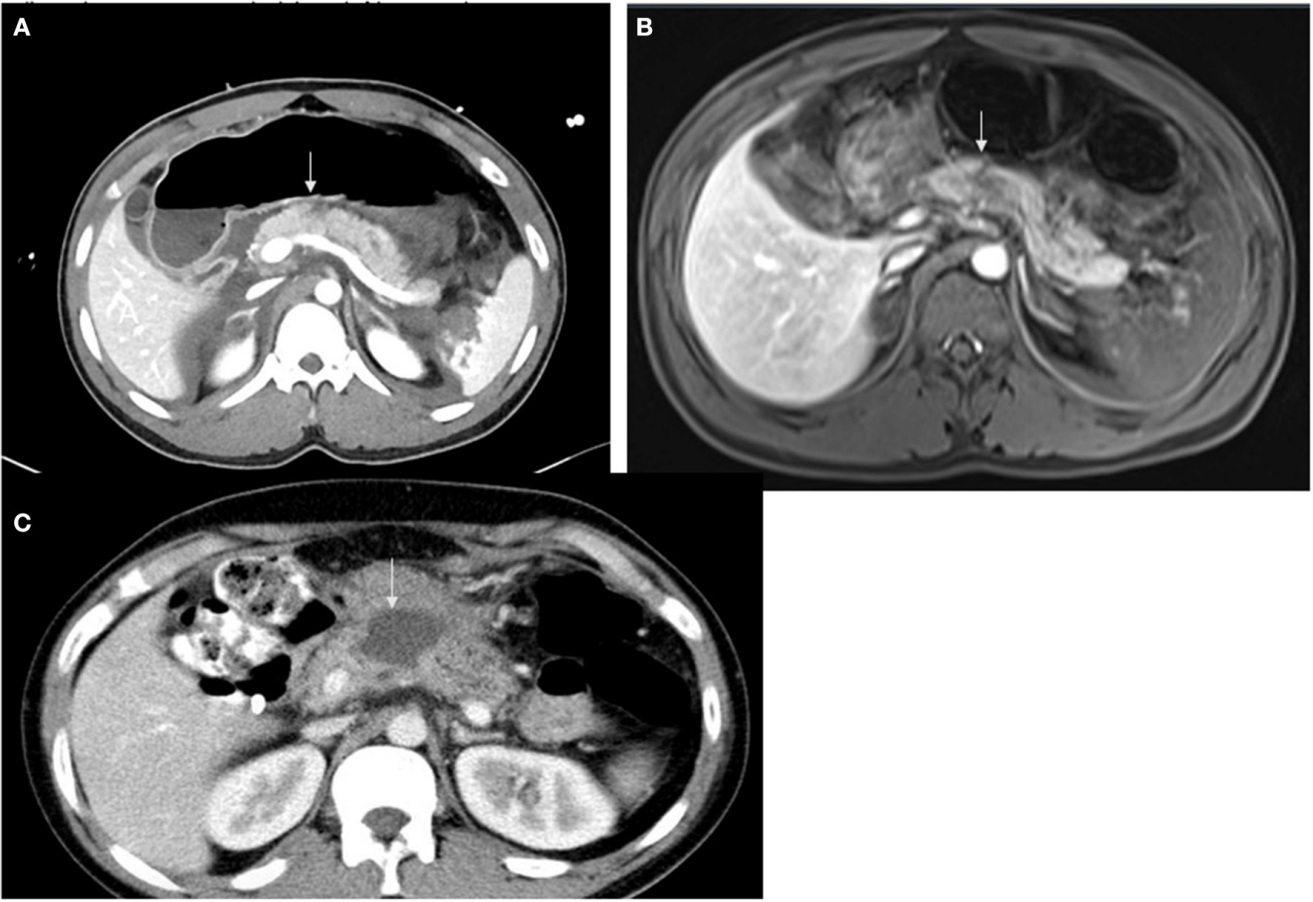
Examples of radiologic findings of pancreatic injury in the study cohort using CT scan and MRI. (A) Axial CT in a patient with pancreatic laceration noted at the superior aspect of the body anterior to the spine involving approximately 50% of pancreatic craniocaudal dimension (white arrows), (B) Axial MRI (same finding) T2: the junction between the head and body of the pancreas shows no enhancement in the dynamic phase representing pancreatic contusion. The pancreatic duct adjacent to the contusion area displays focal dilatation without ductal transection. (C) Axial CT shows pseudocyst.
